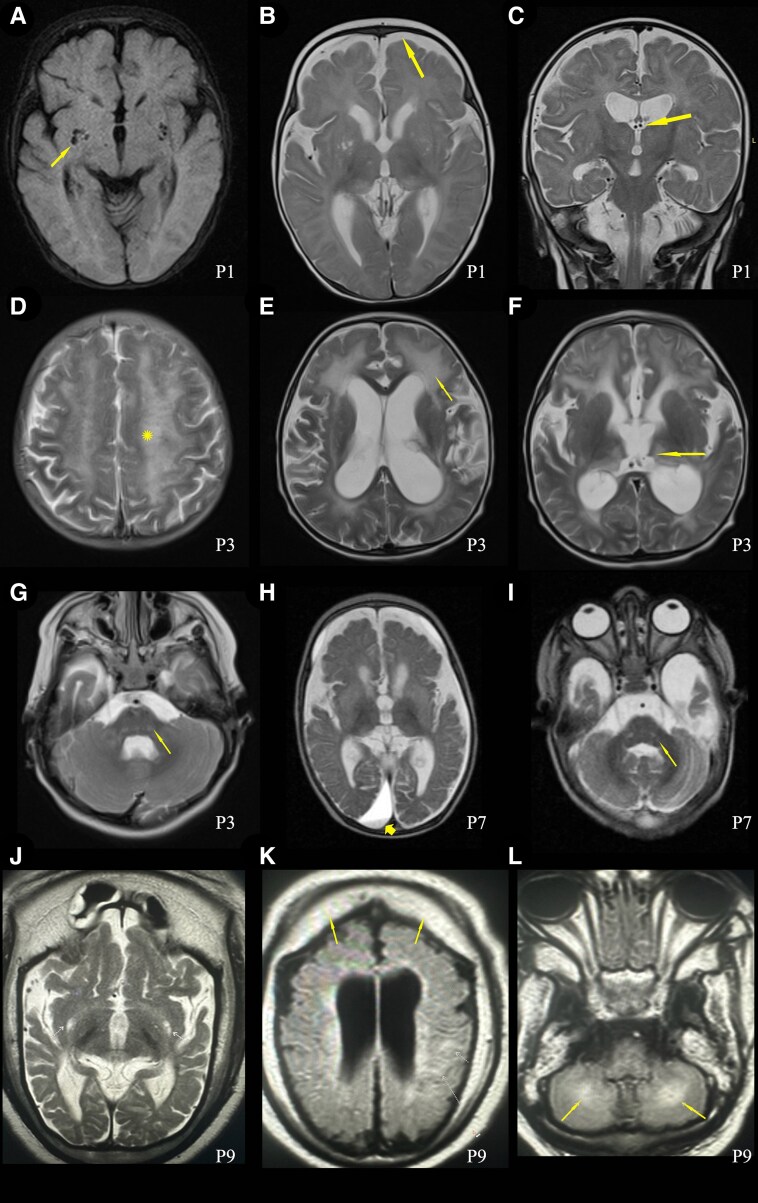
Brain MRIs of cases P1 (A to C), P3 (D to G), P7 (H and I) and P9 (J to L). P1 at 6 months of age: (A) Prominent Virchow-Robin spaces can be seen in the lenticular nuclei and subganglionar area (axial, Fluid attenuated inversion recovery—FLAIR—T2). (B) Enlargement of the ventricular system and extraxial spaces without subdural fluid collections and corpus callosum thinning (axial, Fast spin echo—FSE—T2) (C) High T2 signal intensity in the pulvinar nuclei of the thalami (coronal, FSE T2). Myelination is delayed for a 6-month-old boy. P3 at 3 years of age: (D) symmetrical hyperintensities in bilateral centrum semiovale with a tigroid pattern (axial, T2-weighted); (E) ventriculomegaly, hyperintensity in white matter periventricular and external capsule (T2-weighted axial), (F) loss of volume and abnormal signal in the thalamus (T2-weighted axial), (G) abnormal signal in the posterior pons and bilateral middle cerebellar peduncle (T2-weighted axial). P7 at 9 months of age: (H) Hyperintense signal abnormalities in both the basal ganglia and diffuse brain atrophy prominently at bilateral frontotemporal lobes with secondary ventricular dilatation and frontal bilateral and right occipital subdural hygroma (T2-weighted axial). (I) Hyperintense signal abnormalities are observed in the bilateral brainstem, including severe temporal cortical-subcortical atrophy with significant enlargement of the subarachnoid space of the anterior temporal fossa. P9 at 7 months of age; (J, K) severe frontotemporal cortical-subcortical atrophy with significant enlargement of the subarachnoid space of the bihemispheric convexity associated with frontoparietal subdural hygromas along with compensatory enlargement of the ventricular system and prominent thinning of the corpus callosum, (L) T2-WI hyperintense signal was observed throughout the upper left parietal lobe and medial cerebellar hemispheres (arrows).
